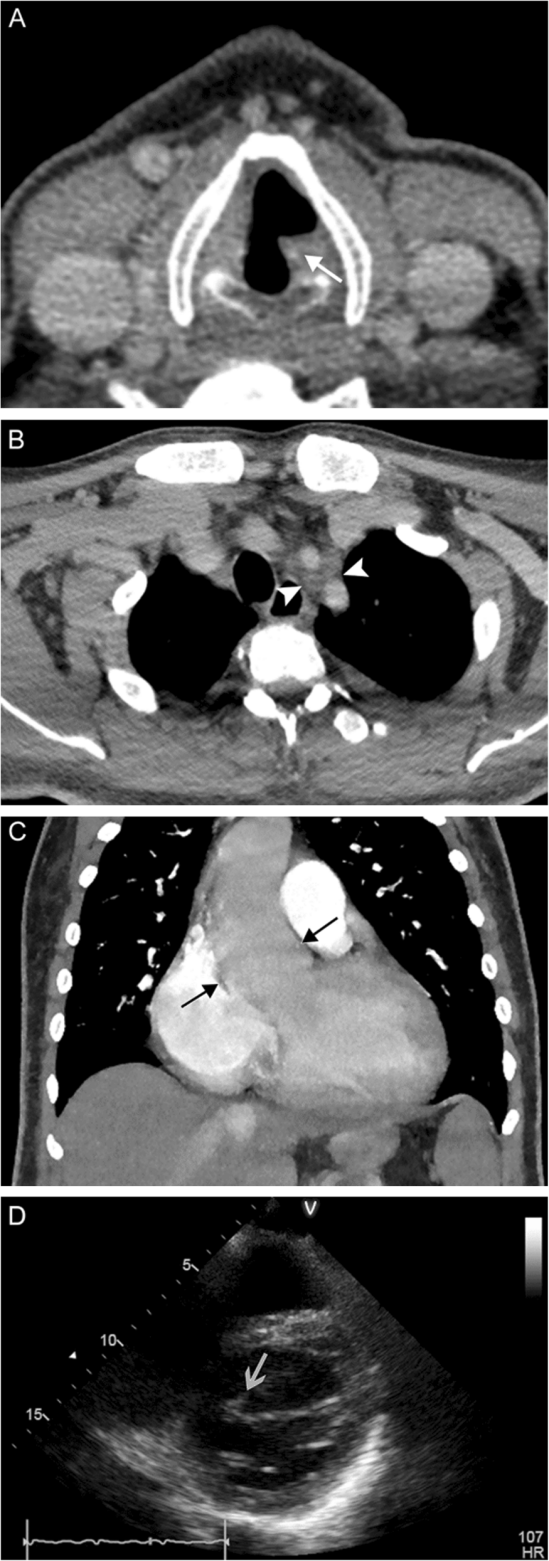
Endocarditis. A 41-year-old previously healthy man presents with intermittent hoarse voice and unexpected weight loss. Axial contrast-enhanced CT images through the larynx (a) and mediastinum (b) reveal left vocal fold rotation (white arrow) and lymphadenopathy surrounding the left carotid and subclavian arteries (white arrowheads). A coronal CTA image (c) reveals an ascending aortic aneurysm (black arrows). Echocardiogram (d) reveals a bicuspid valve with vegetations (gray arrow) and severe aortic stenosis. Overall, findings are consistent with compression/stretching of the left recurrent laryngeal nerve due to reactive lymphadenopathy and a dilated aortic arch in the setting of endocarditis
